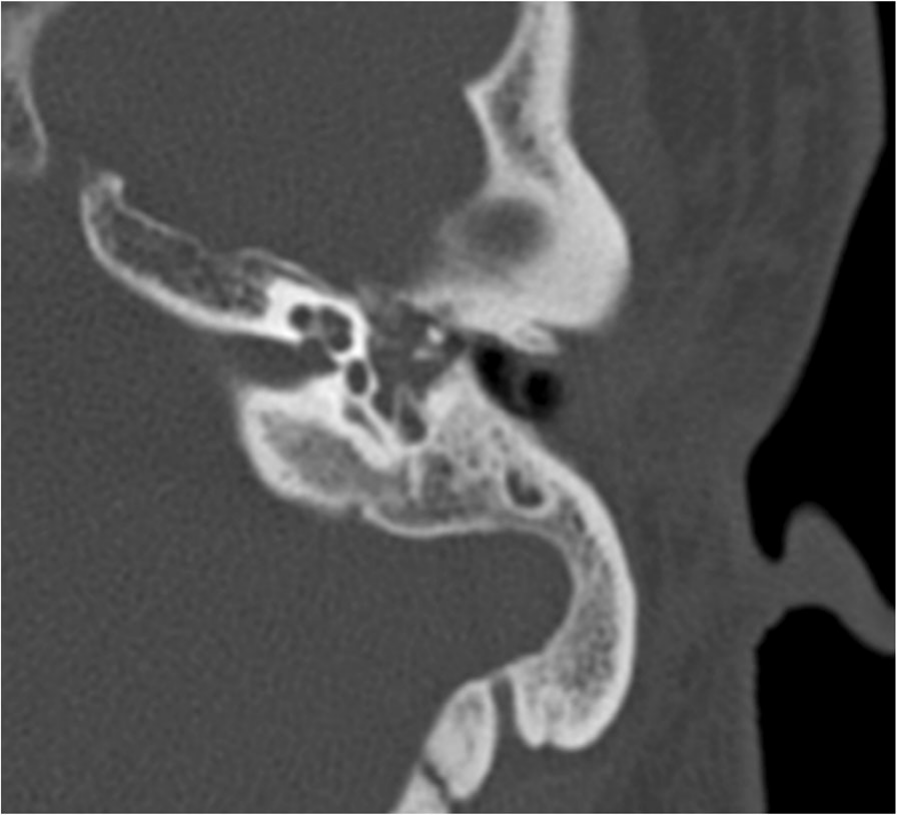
Axial reconstruction of a temporal bone CT, at the level of the ossicular chain, of a 36-year-old Down syndrome patient demonstrating hypopneumatization of the mastoid and signs of left middle chronic otitis, with an erosion of the ossicular chain, namely the stapes
